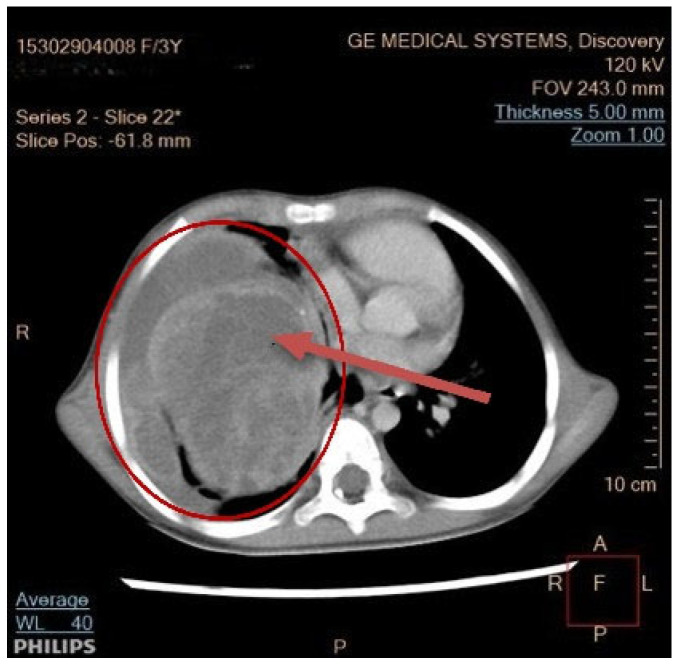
Enhanced contrast of CT scan. The arrow indicates a large 8 × 9 × 16 cm heterogeneously enhancing solid mass in the right hemithorax with pleural effusion.
